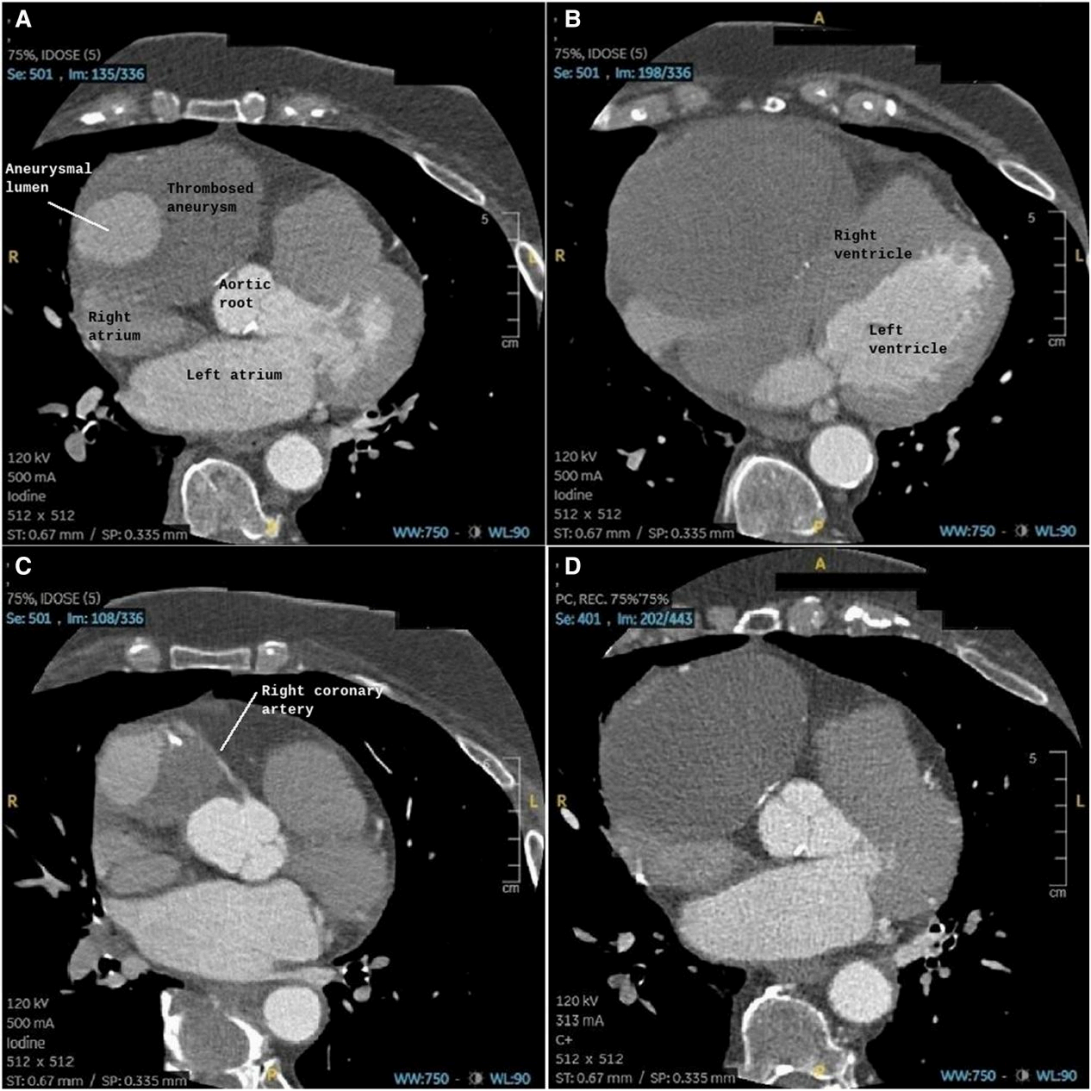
Axial coronary CTA showing a mass adjacent to the right coronary artery, suggestive of a coronary aneurysm, before (*A*) and after (*B*) percutaneous exclusion. Note the absence of contrast enhancement within the mass after exclusion.
